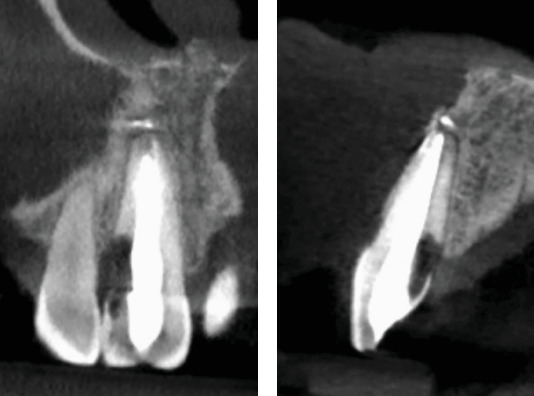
The (a) axial and (b) sagittal CBCT sections at 20 years allow us to visualize the extent of the lesion.
